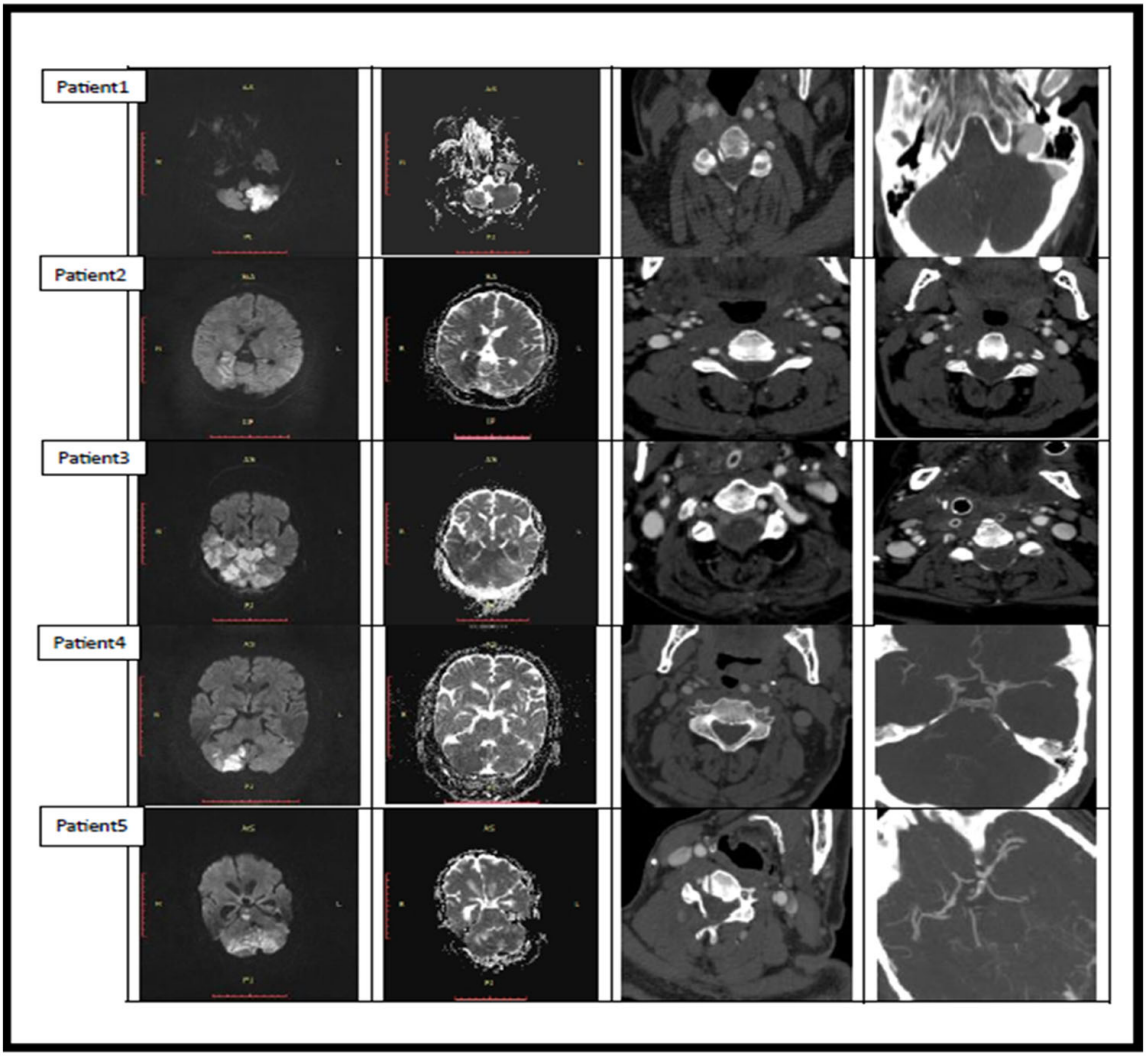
Diffusion‐weighted magnetic resonance imaging, apparent diffusion coefficient, and computed tomography angiography images in the patients of this study show acute infarction, sometimes multiple in the area of posterior cerebral artery blood supply and fetal type variants, hypoplasia of the vertebral arteries and basilar artery, and dissection in the vertebral artery.
